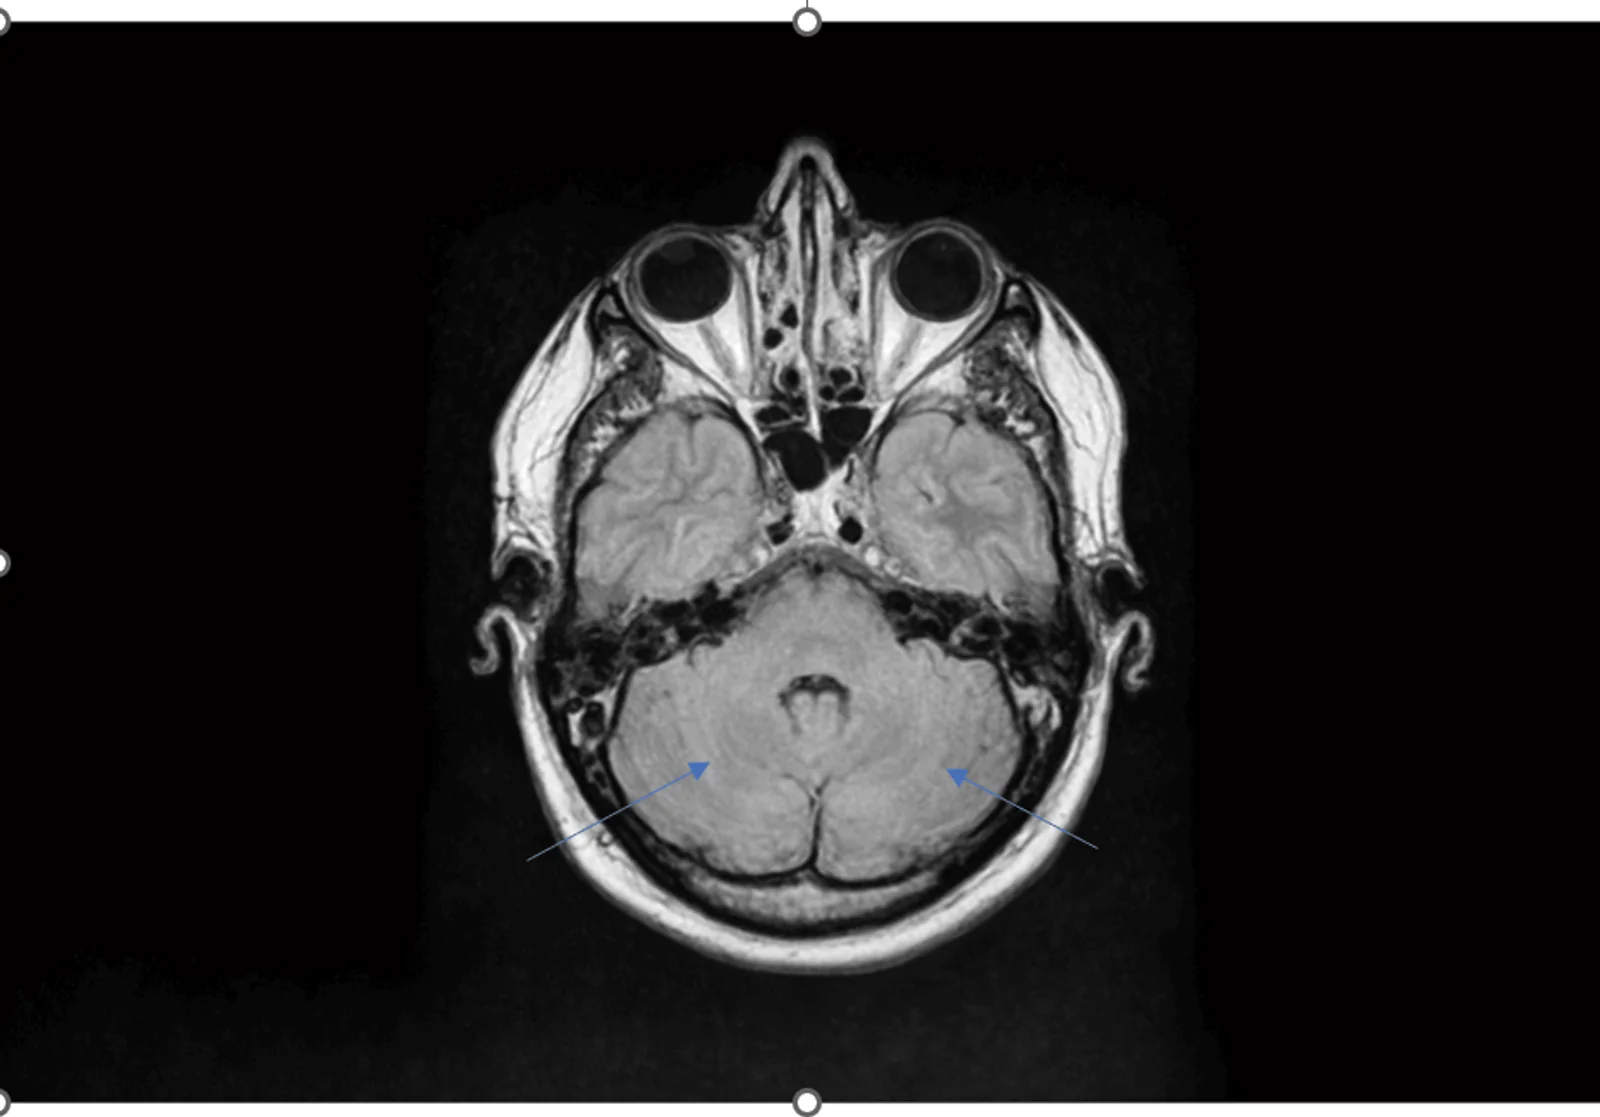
T1 brain MRI (magna (axial section)). Blue arrows identify the bilateral cerebellar hemispheres The brain MRI shows normal brain structure of bilateral cerebellar hemispheres with no lesions or atrophy identified.
